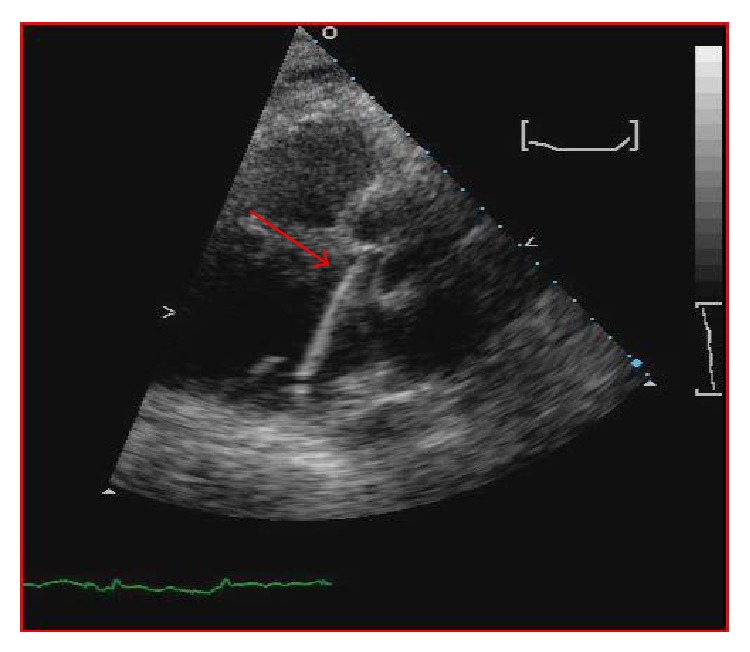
Parasternal long axis view showing the Impella catheter tip resting on the inferior basal wall (red arrow).
